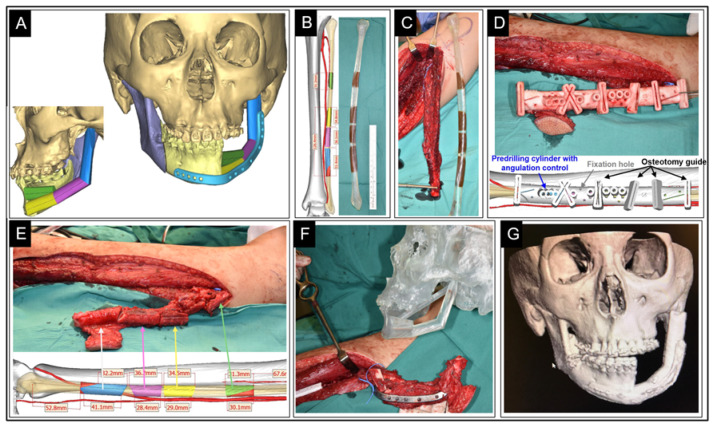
Three-dimensional printing in free fibula flap mandible reconstruction following odontogenic tumor resection: (A) Computer (CAD) plan of a reconstructed mandible; (B) Computer model and 3D print of fibula with planned construct segments marked out; (C) 3D-printed model next to actual patient’s fibula in vivo; (D) 3D-printed jig with osteotomy (cutting) guides on fibula; (E) segmented fibula in vivo and computer model with corresponding segments labeled; (F) assembled construct fixed with pre-bent metal reconstruction plate, prior to division of vascular pedicle; (G) CT image of patient three months following surgery. (Images courtesy of Dr. Chew Khong-Yik, Department of Plastic Surgery, Singapore General Hospital, Singapore).
